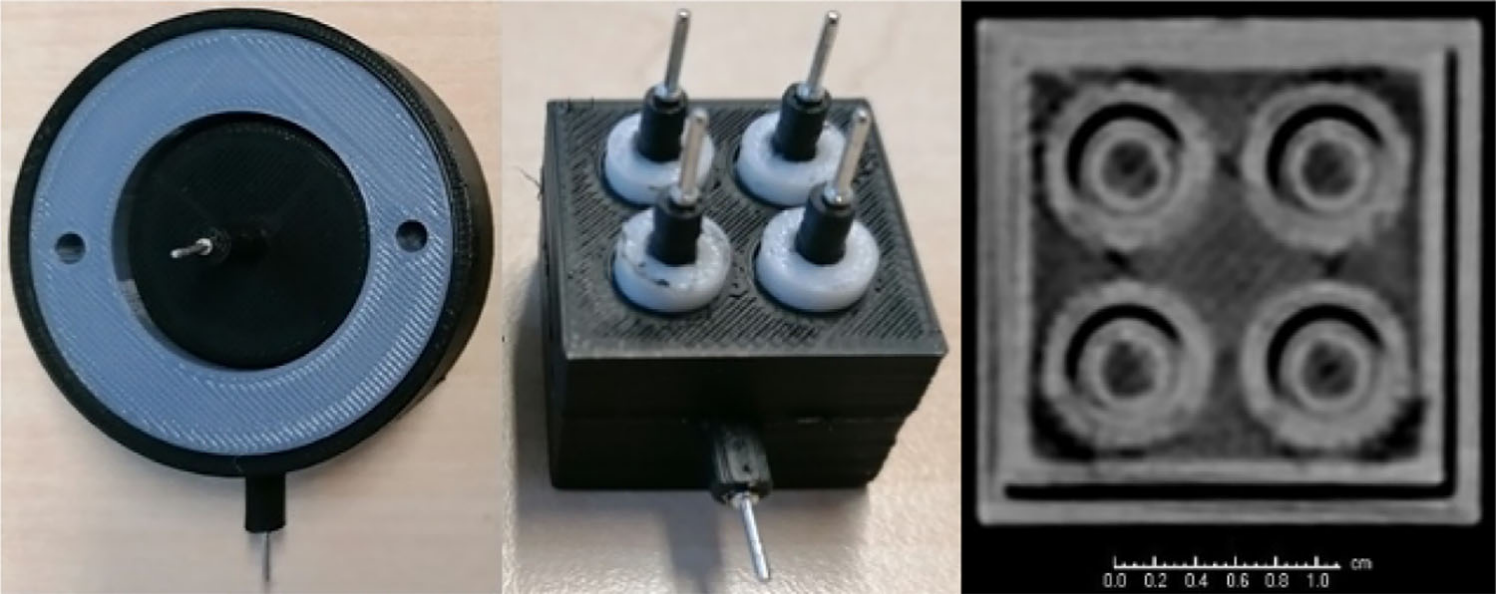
Photos of FC fabricated with additive manufacturing. Left: Photo of the modular large‐volume detector (30 mm/56 mm inner/outer diameter, 100 mm height). Middle/right: Photo/transversal x‐ray CT of the 2×2 array (25 mm/17 mm width/height of black cube). FC, Faraday‐cup.
